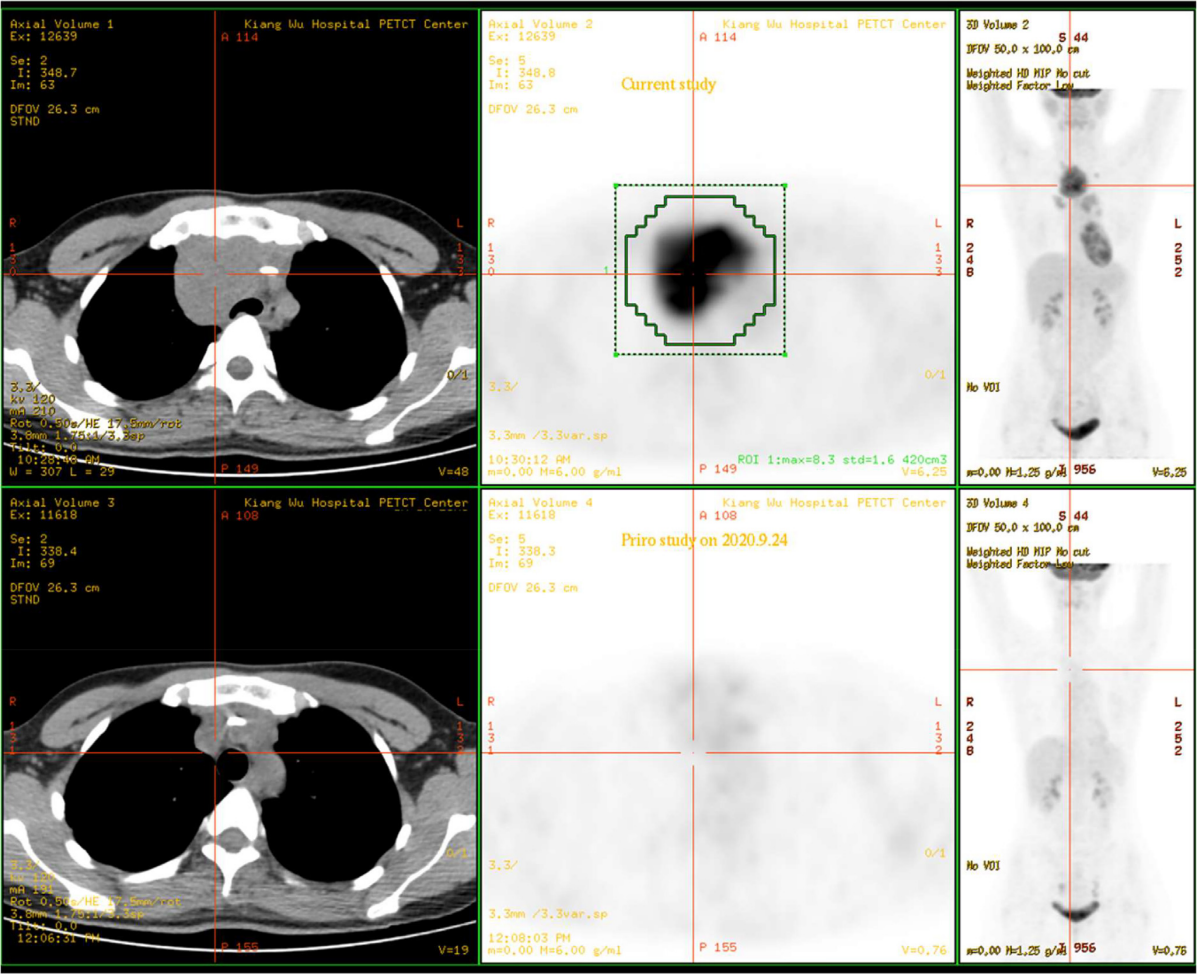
The ^18^F-FDG PET/CT scan showed relapsed disease in the mediastinal region. The ^18^F-FDG PET/CT scan on April 07, 2021 showed recurrent tumor with hypermetabolism in the mediastinum after half a year.
